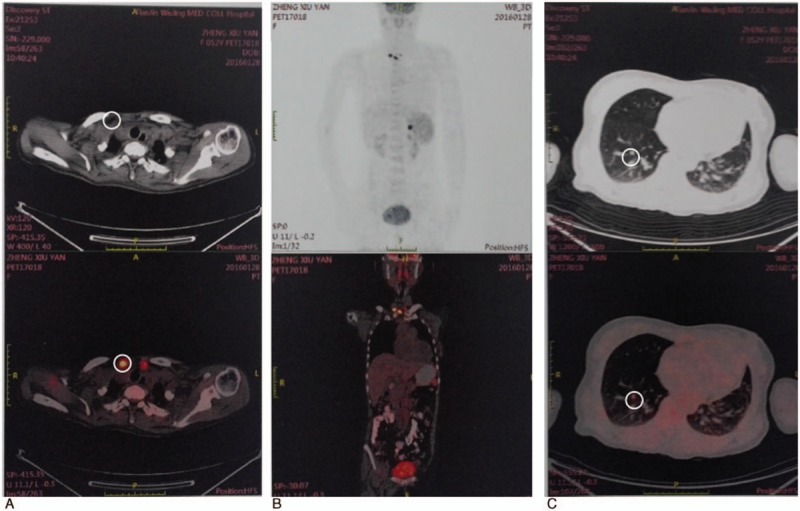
PET/CT scans. A and B, ^18^F-FDG PET/CT showed lymph metastase in the suprasternal suprasternal fossa. C, ^18^F-FDG PET/CT showed multifocal nodules in lung. PET/CT = positron emission tomography/computer tomograph.
